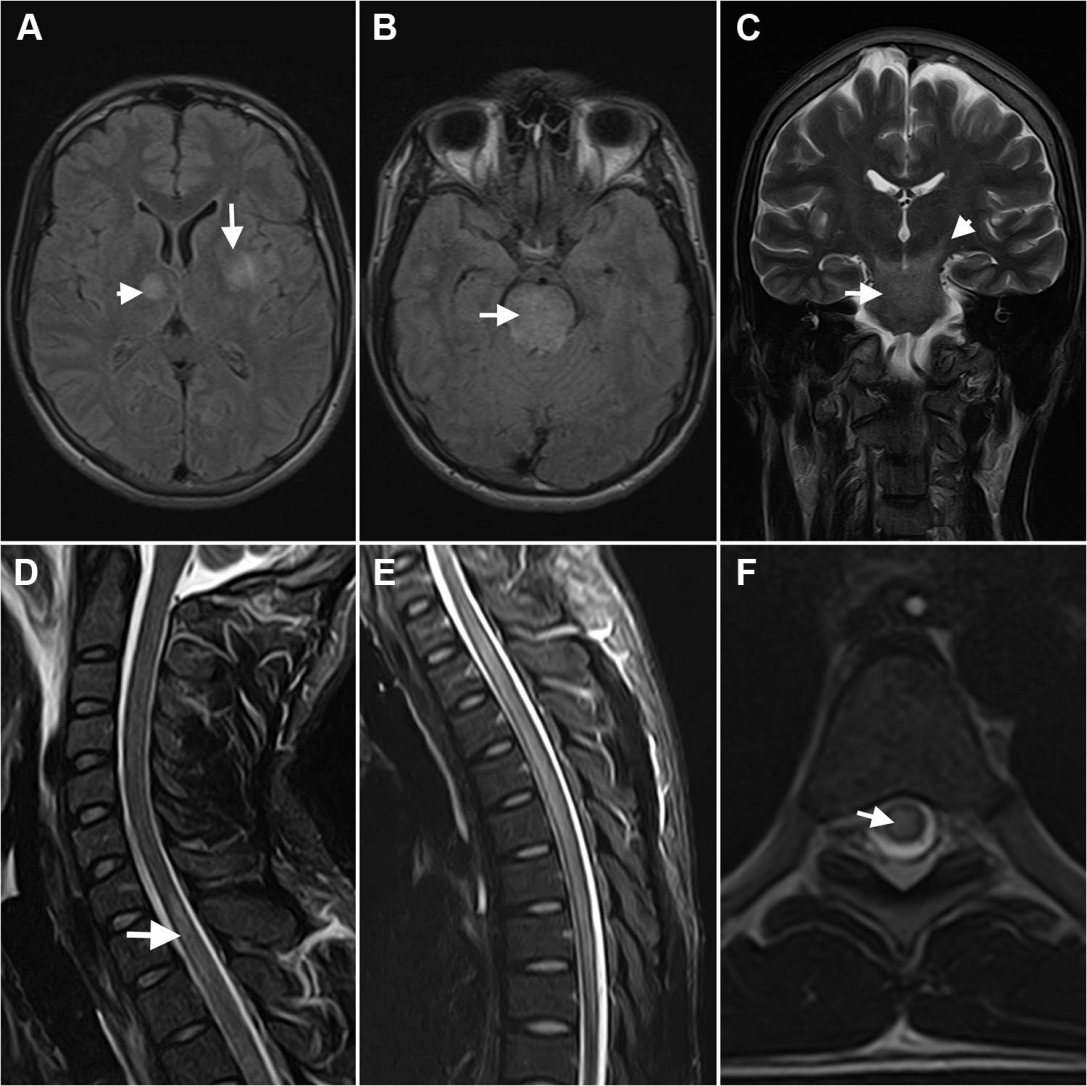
Brain MRI. A Axial FLAIR showing hyperintensities in the right internal capsule (arrowhead) and left basal ganglia (arrow); B axial FLAIR showing diffuse hyperintensity of the pons (arrow); C coronal T2 with extensive hyperintensities of internal capsules (arrowhead), midbrain, and pons (arrow). Spinal cord MRI. D Sagittal STIR showing hyperintensity below C7 level of the spinal cord; E longitudinally extensive hyperintensity along the entire thoracic spinal cord; F axial T2 with transverse hyperintensity of the spinal cord (arrow)
